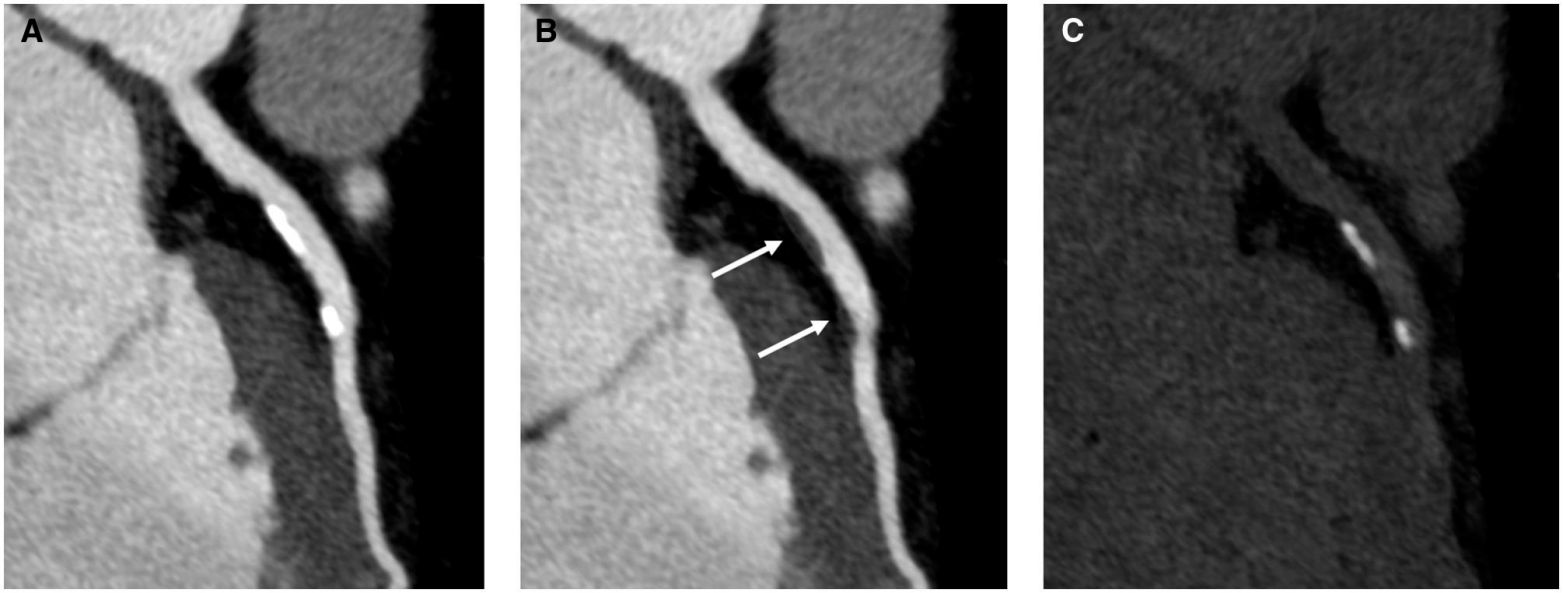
A 53-year-old male patient with increased cardiovascular risk profile and recurrent episodes of atypical chest pain undergoing dual-source photon-counting detector CT. Curved planar reformations of conventional (A), virtual non-calcium (B), and virtual non-contrast (C) images show 2 calcified plaques in the proximal left anterior descending (LAD) artery (arrows). Note the dual-energy-based removal of calcium (arrows) in the virtual non-calcium and the dual-energy-based removal of iodine in the virtual non-contrast image.
